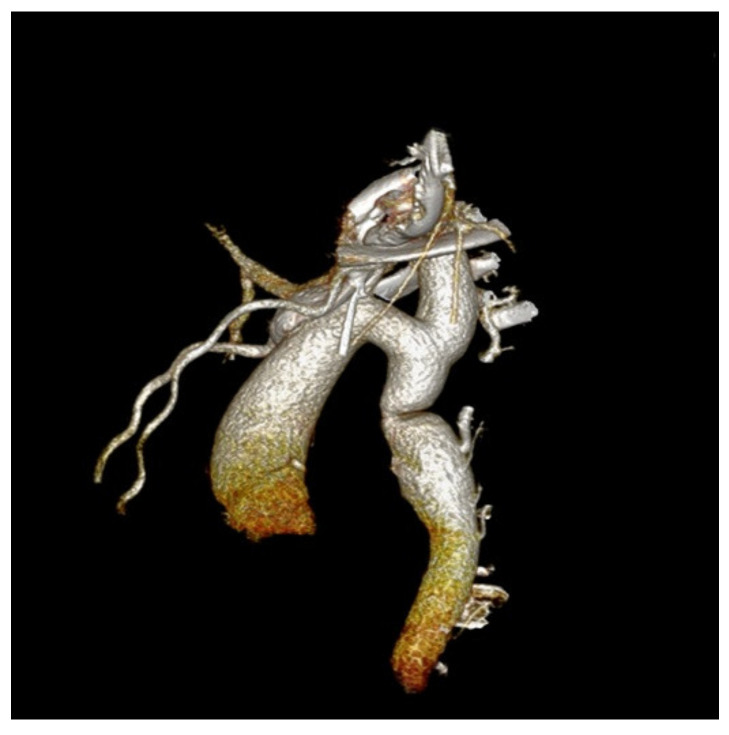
Angio-CT examination of the aorta precisely located the obstruction—a focal narrowing area, multiple collateral arteries, and bilateral dilated intercostal and subclavian arteries.
